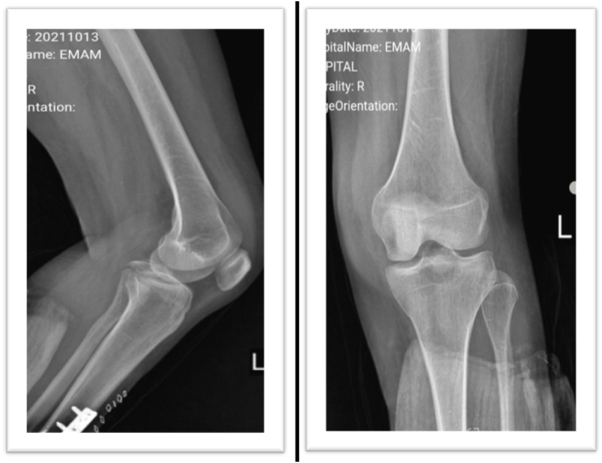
Legs’ front view and side view radiography after follow-up.
